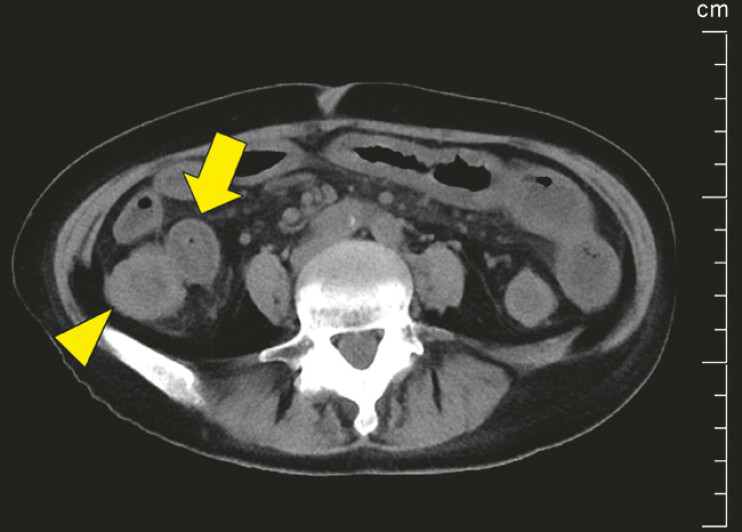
Abdominal computed tomography showed wall thickening (8 mm) in the ascending colon (arrow) and terminal ileum (arrowhead), typical of diffuse edema and wall thickening observed in neutropenic enterocolitis.
